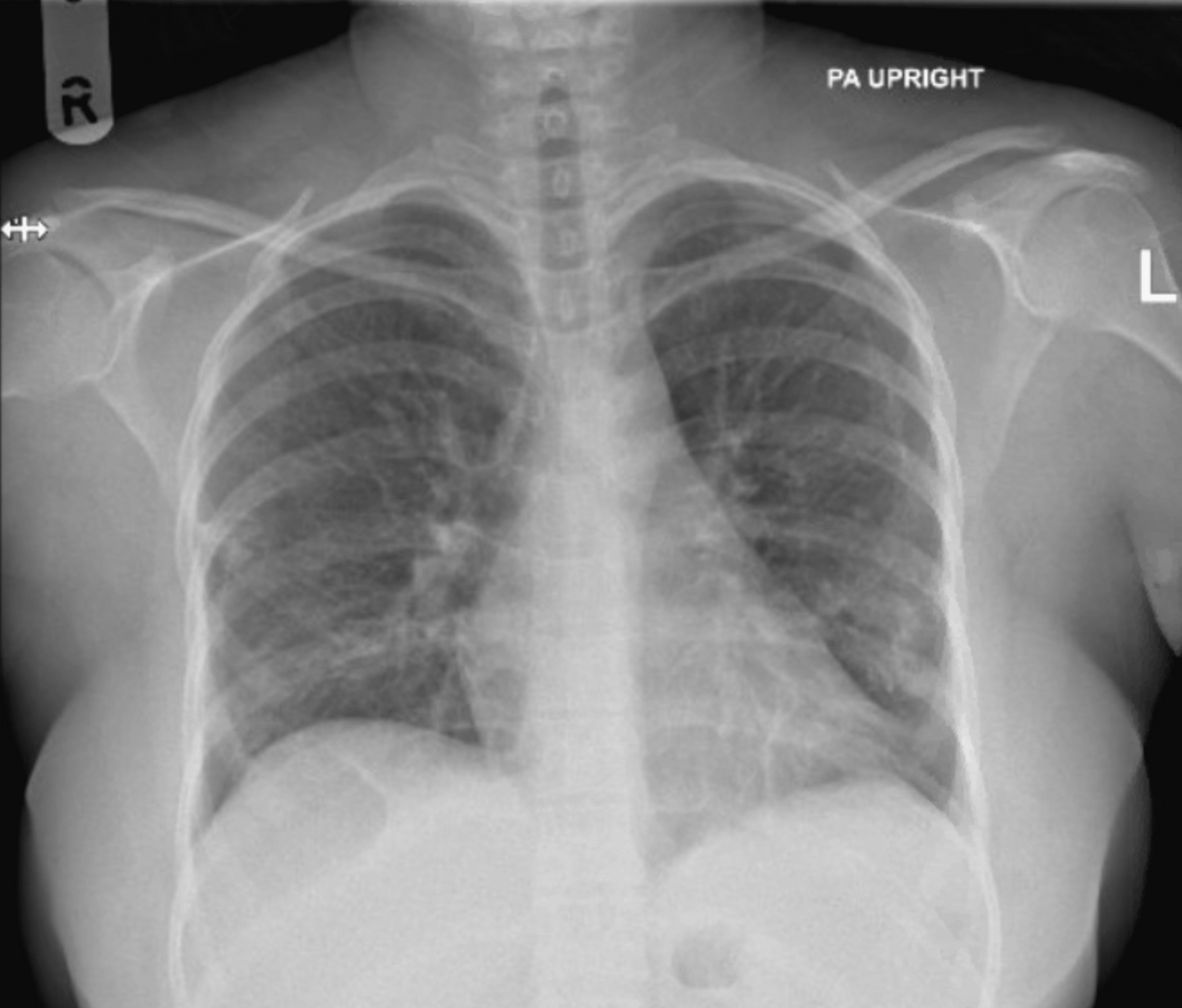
Chest X-ray showing a normal lung parenchyma
